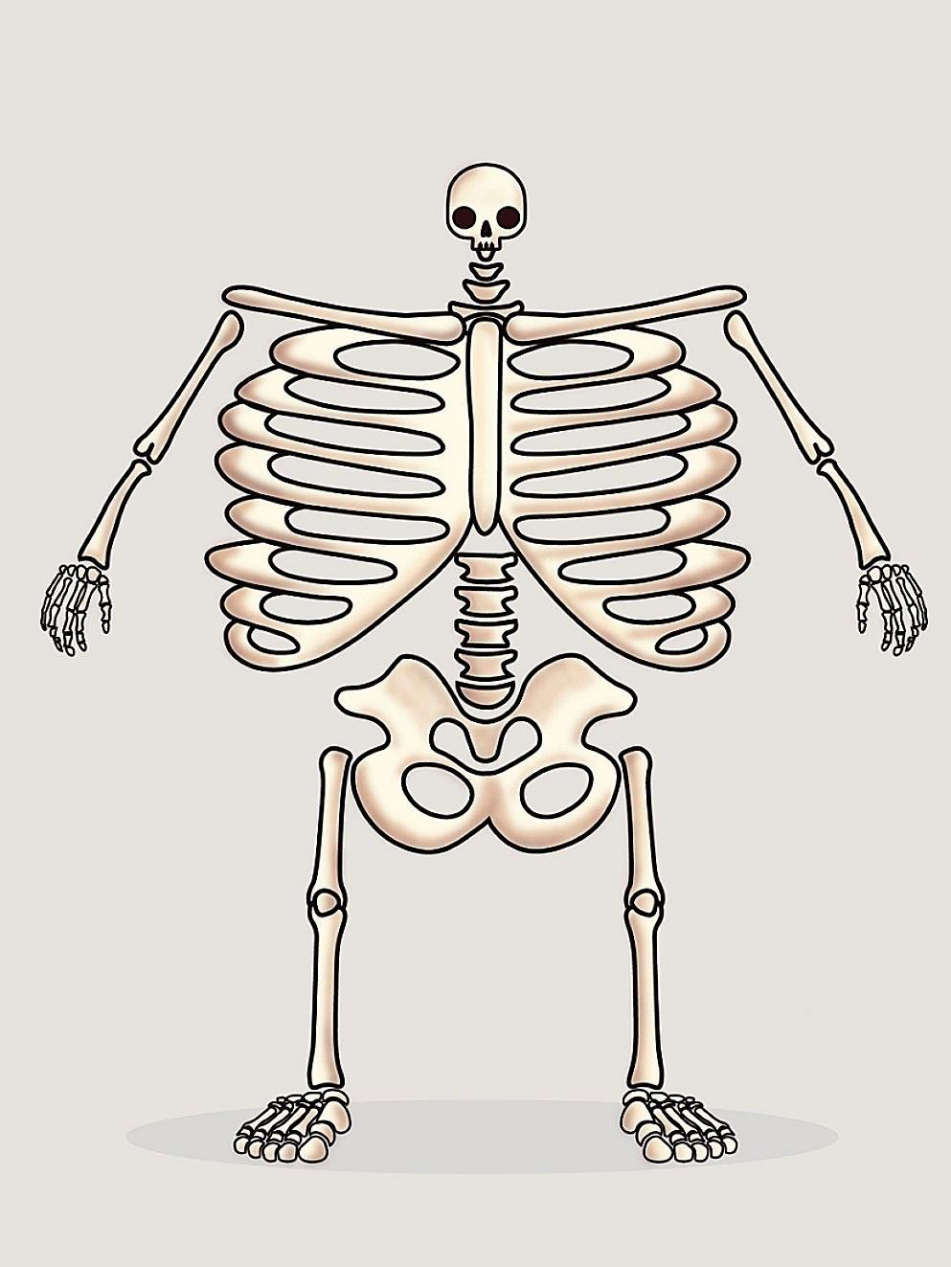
The [^18^F]PSMA-1007 UBUs homunculus. This figure visually represents [^18^F]PSMA-1007 UBUs’ distribution across the human skeleton, emphasizing high-incidence areas with scaled prominence to underscore their clinical significance
